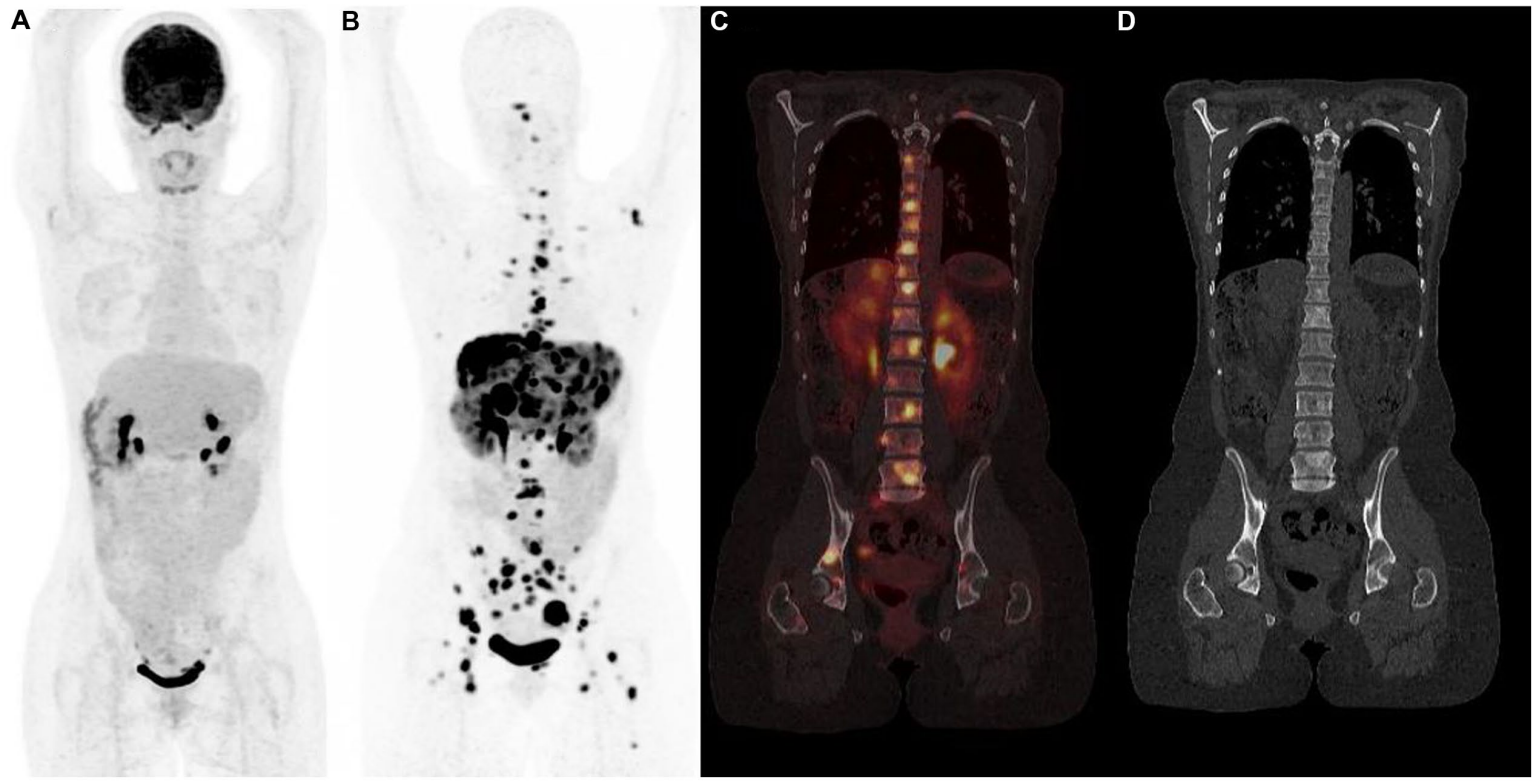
^18^F-FDG (A) and ^68^Ga-DOTATOC (B–D) PET/CT results (anterior MIP, coronal PET/CT, coronal CT) in a 48 years-old woman with grade 2 metastatic pancreatic neuroendocrine tumor showing multiple bone sclerotic metastases characterized by intense and pathologic ^68^Ga-DOTATOC uptake. ^18^F-FDG PET/CT failed to detect metastatic spread.
